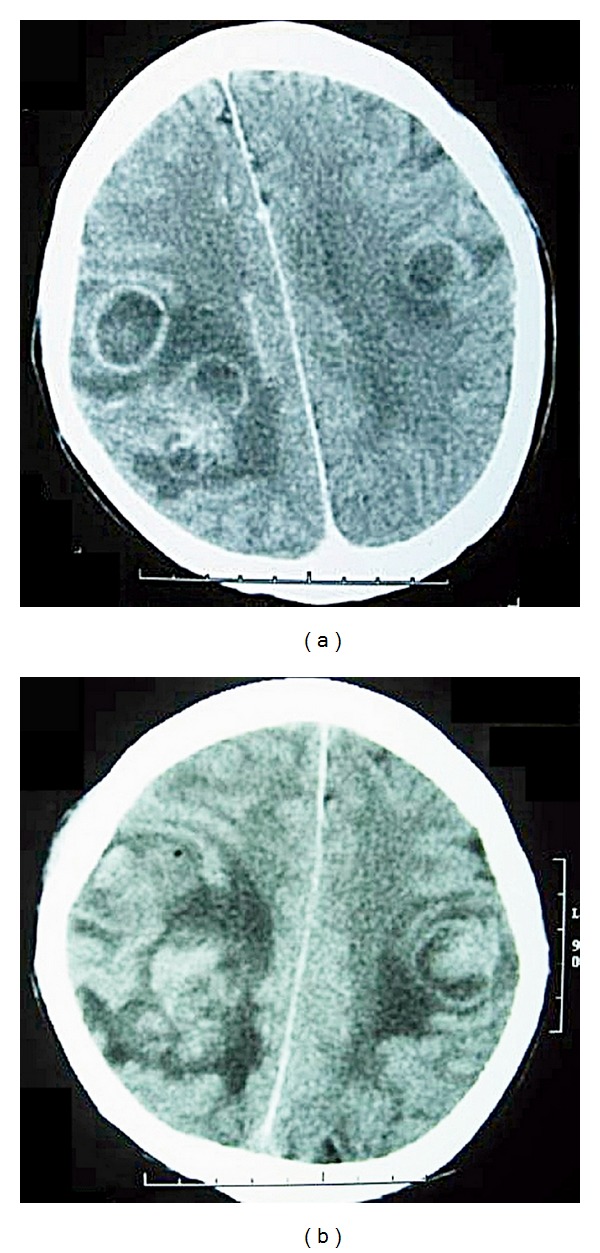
(a) Brain CT with contrast enhanced showed multiple solitary abscesses over right temporal and bilateral frontal, parietal area with brain edema on April 2, 2002. (b) Brain CT on April 9, 2002, found severe brain edema even under the mannitol and dexamethasone use.
